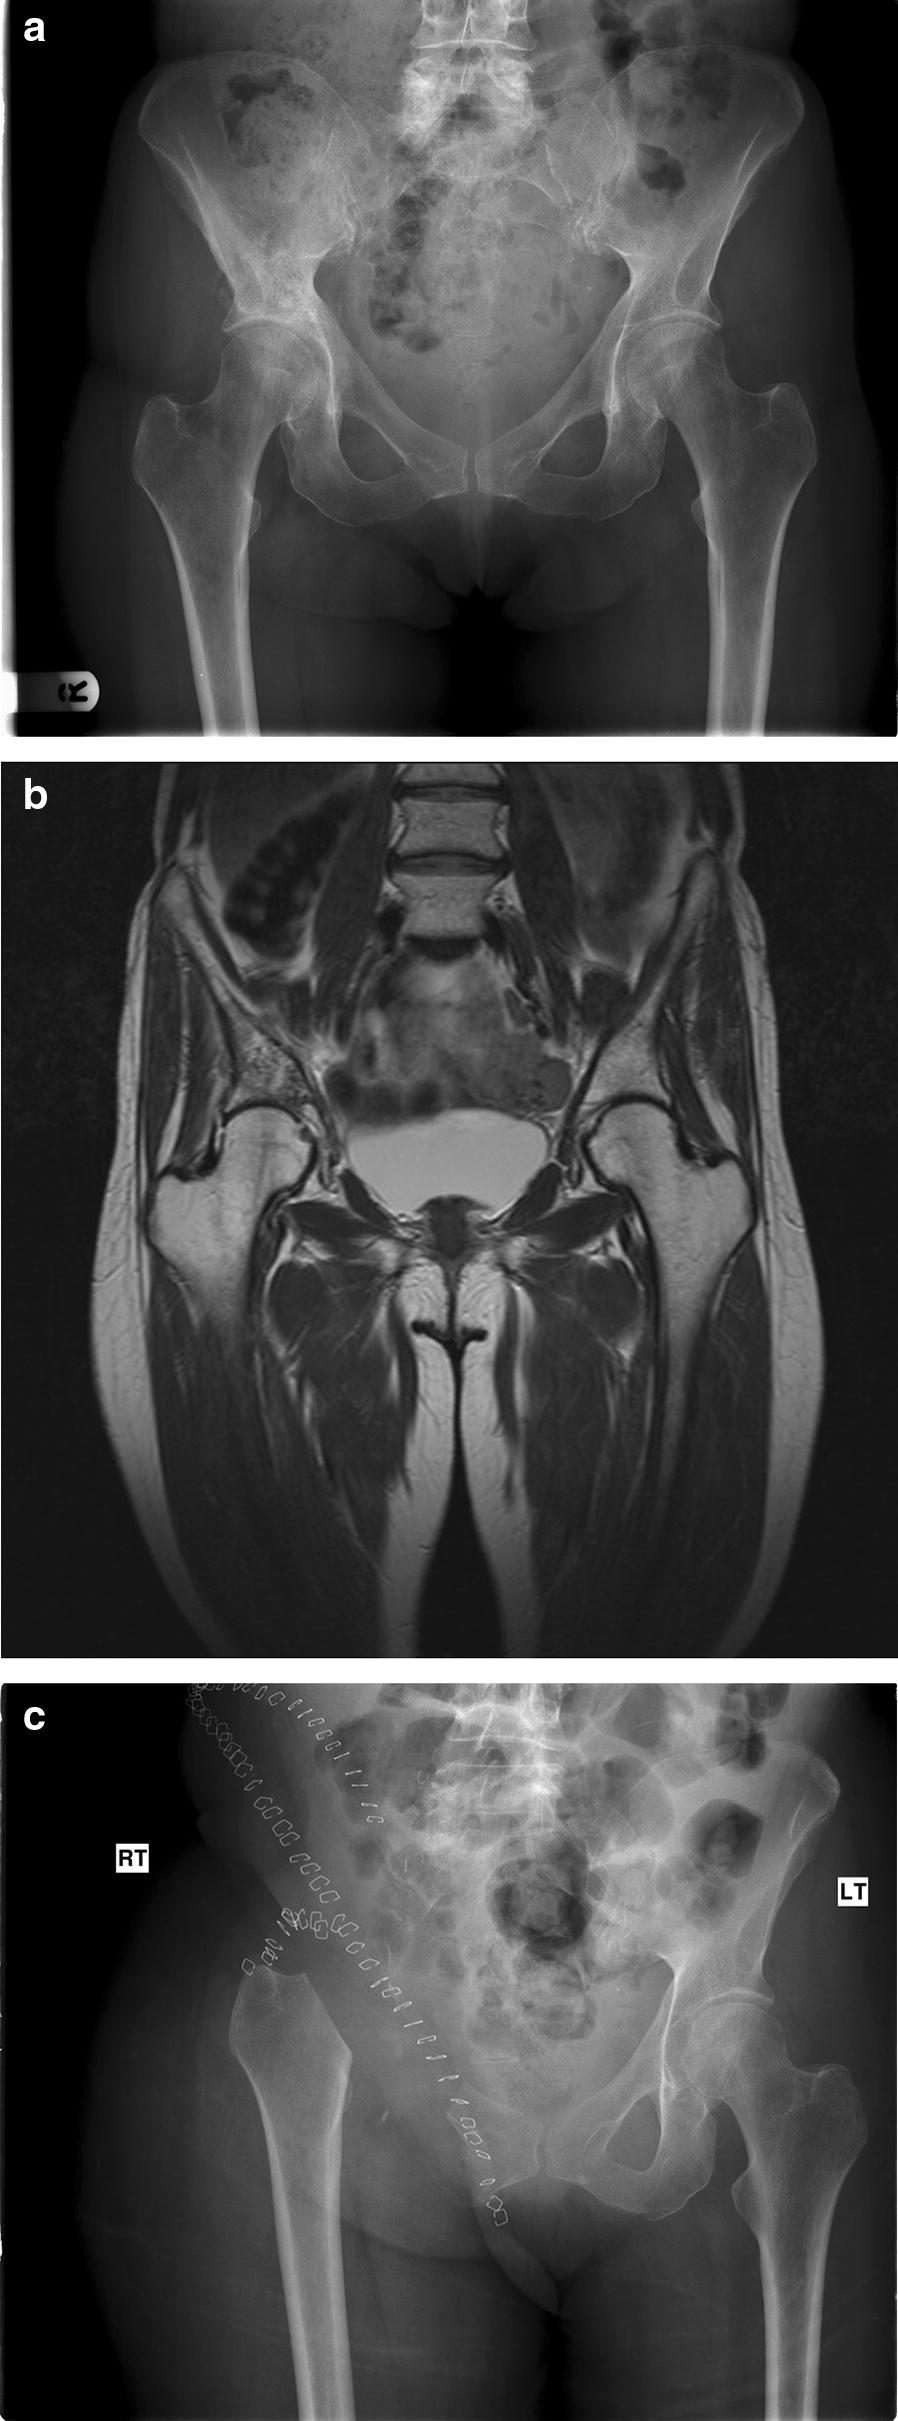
Pre-operative pelvis radiograph (a) and MRI (b) and post-operative radiograph (c) of a patient who underwent LSS through an internal hemipelvectomy and no reconstruction (flail hip) with wide margins (> 20 mm) and long-term survival achieved
